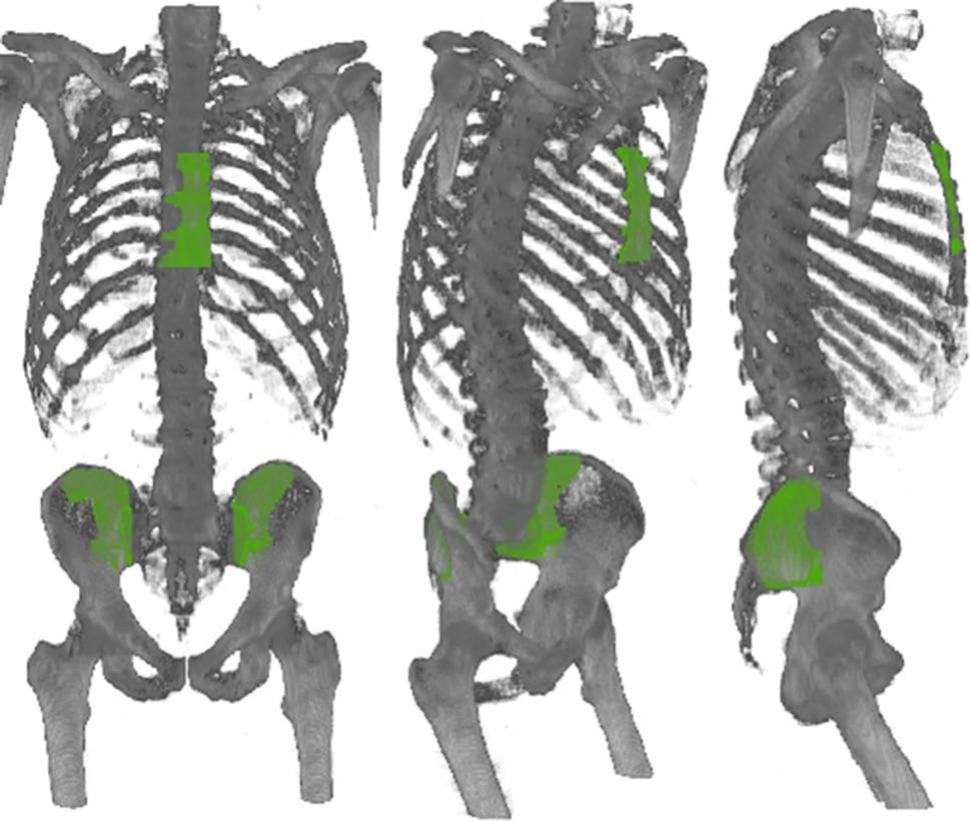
Segmentations of the bone marrow in biopsy locations (Sternum, Left Posterior Iliac Crest and Right Posterior Iliac Crest) represented in green overlays with the 3D reconstruction of a CT image
